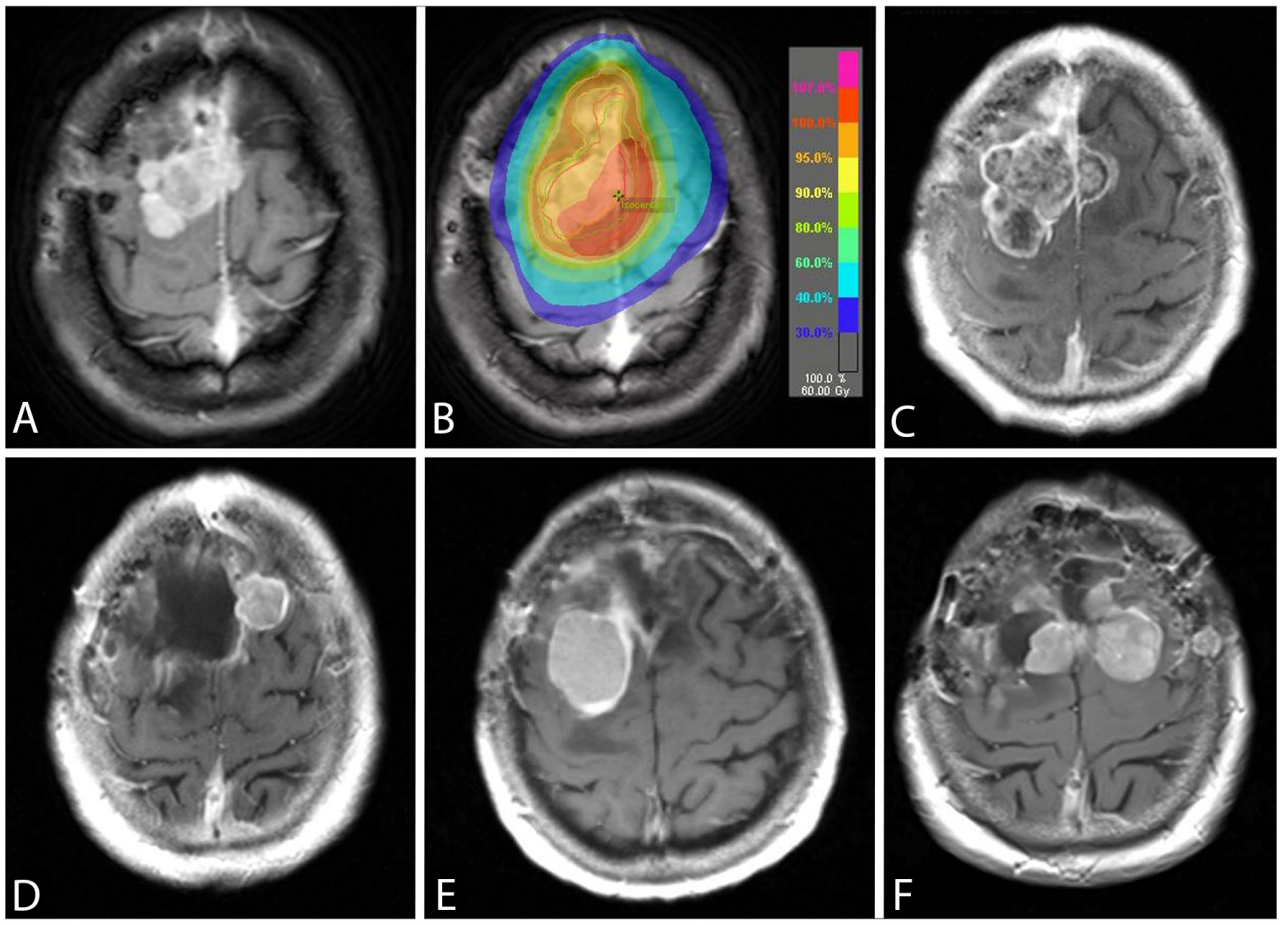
An axial post-gadolinium T1-weighted image showing the *TERTp* wild-typeconvexity WHO grade II meningioma (case Pat08) (A). A radiation therapy with 60 Gy was applied after a subtotal tumor resection (B) and the patient was progression-free for 12 months. The follow-up MRI showed a progressive tumor (C) and the pathological diagnosis was consistent with an anaplastic meningioma WHO grade III with a new developed *TERTp* mutation. While the recurrence in (D) was proven to be *TERTp* wild-type, the subsequent recurrence (E) was *TERTp*-mutant. At the time of last surgery (F), the *TERTp* mutation was detected in the right-sided, but not in the left-sided meningioma. The patient passed away 22 months after the first emergence of *TERTp* mutation due to progressive disease.
